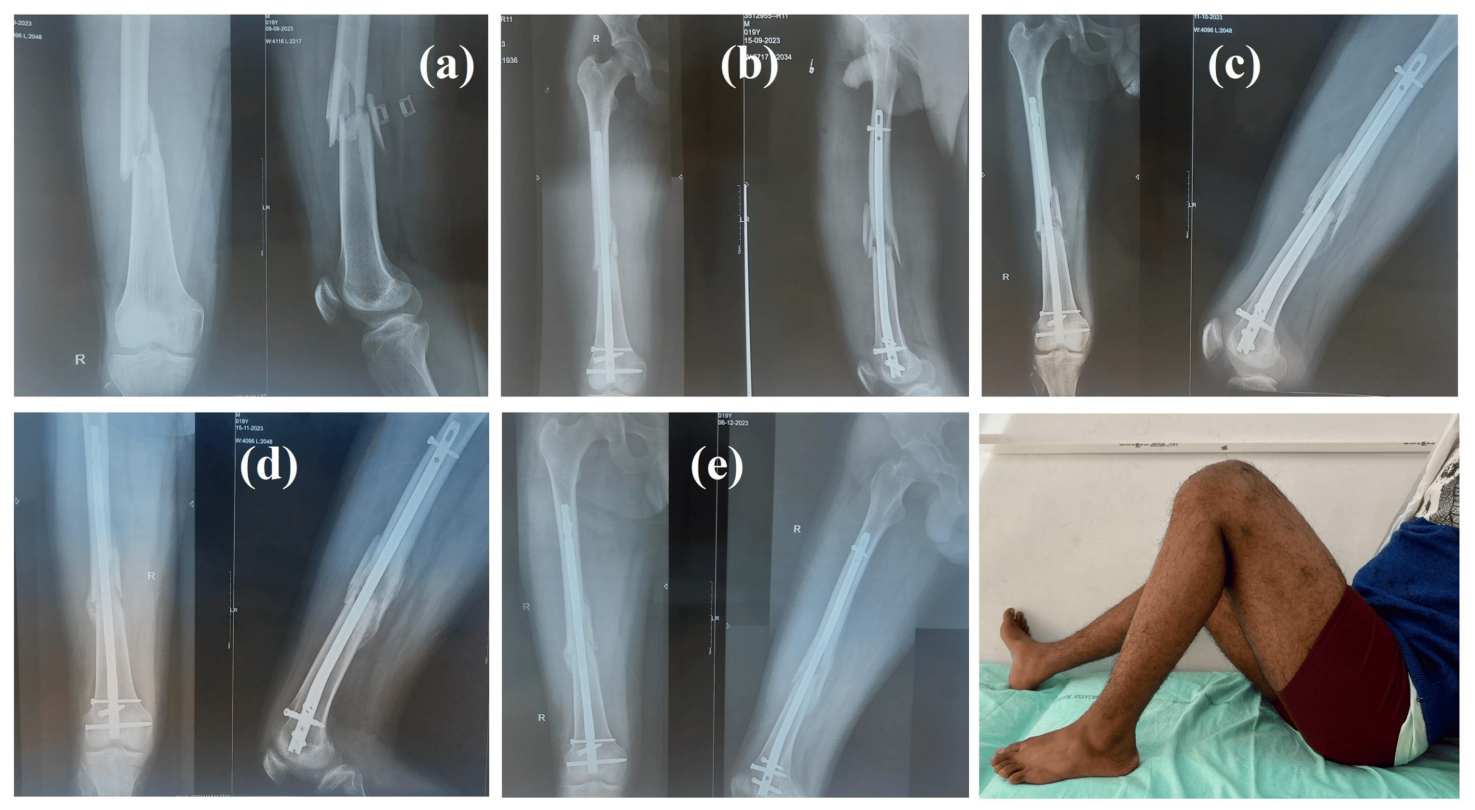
Serial radiographs of a distal third femur fracture treated with DFN-ML (a) Preoperative radiograph showing distal third femur fracture. (b) Immediate postoperative radiograph (POD 1) following DFN-ML fixation. (c) Follow-up at four weeks. (d) Follow-up at eight weeks. (e) Follow-up at 12 weeks demonstrating progressive healing and maintained fixation stability. DFN-ML: distal femur nail multilocking; POD: postoperative day
